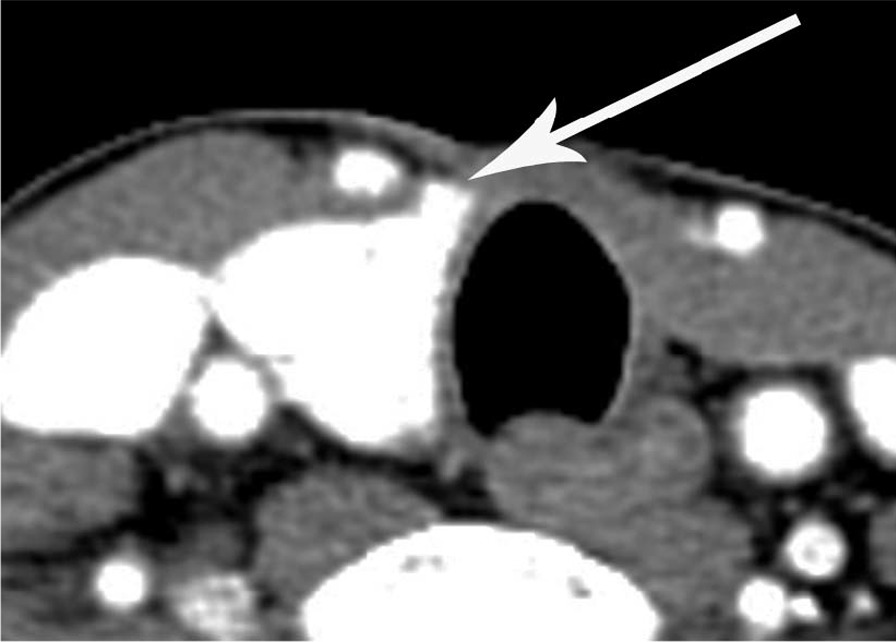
A 61-year-old woman with left hemithyroidectomy for papillary thyroid cancer. Axial contrast-enhanced CT scan shows the absence of isthmus and blunt edge of medial end of remnant thyroid gland (arrow). The angle of the medial end of remnant thyroid gland was measured as − 36°
